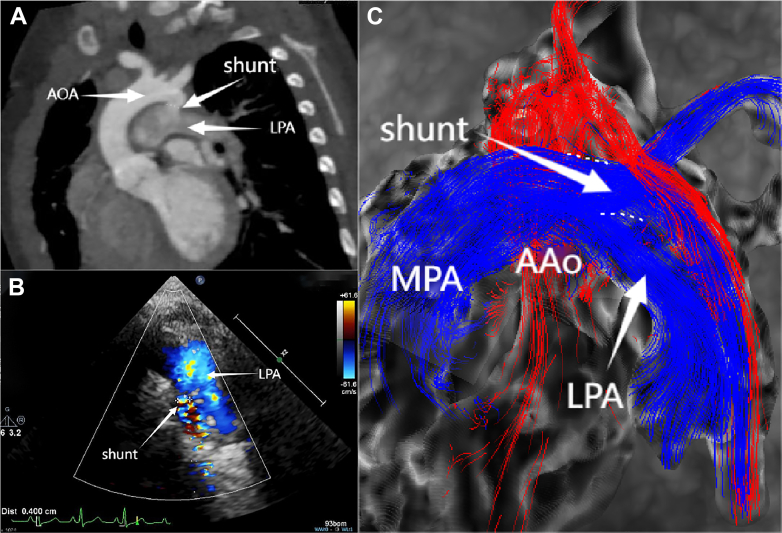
Evaluation of the Size and Direction of the Reversed Potts Shunt Using 3 Imaging Modalities (A) Cardiac computed tomography imaging showing the location and size of the RPS. (B) Echocardiography showing a shunt size of approximately 0.4 cm with bidirectional flow, predominantly right to left (blue flow indicates right-to-left shunting). (C) Cardiac magnetic resonance shows streamline visualization of systemic and pulmonary circulation, with blue indicating pulmonary artery flow and red indicating aortic flow, and pulmonary artery flow is seen entering the descending aorta through the shunt. AAo = ascending aorta; AOA = aortic arch; LPA = left pulmonary artery; MPA = main pulmonary artery; shunt = reversed Potts shunt.
